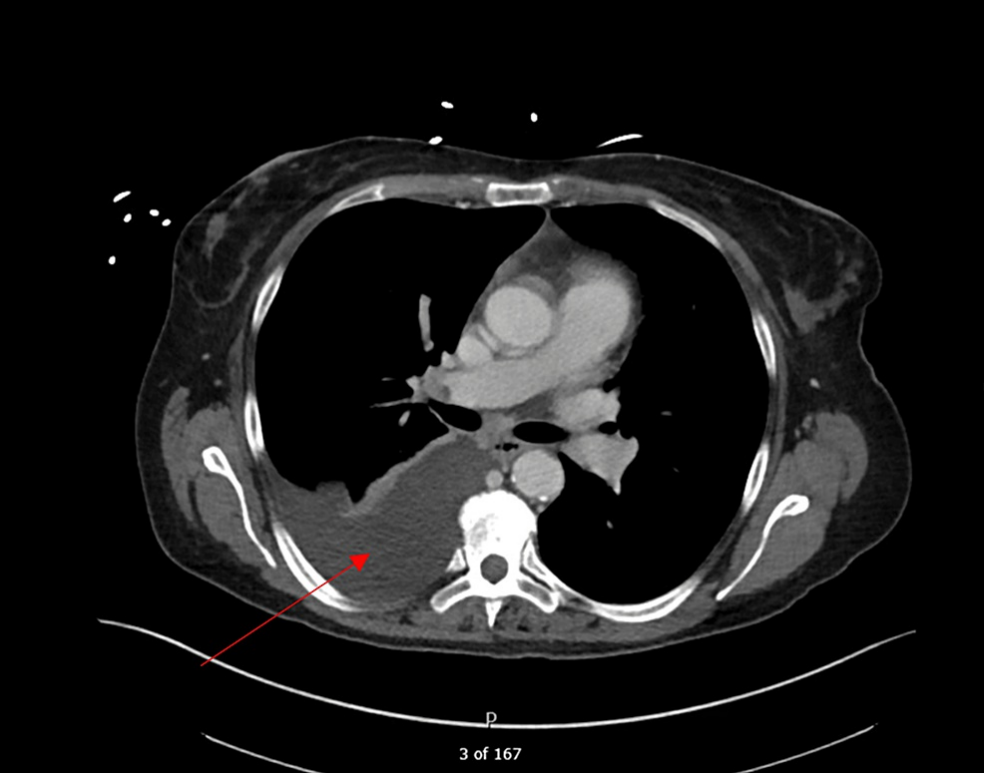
Moderate right pleural effusion (indicated by arrow) visualized on CT scan upon patient developing right-sided basilar crackles on day five of her admission
